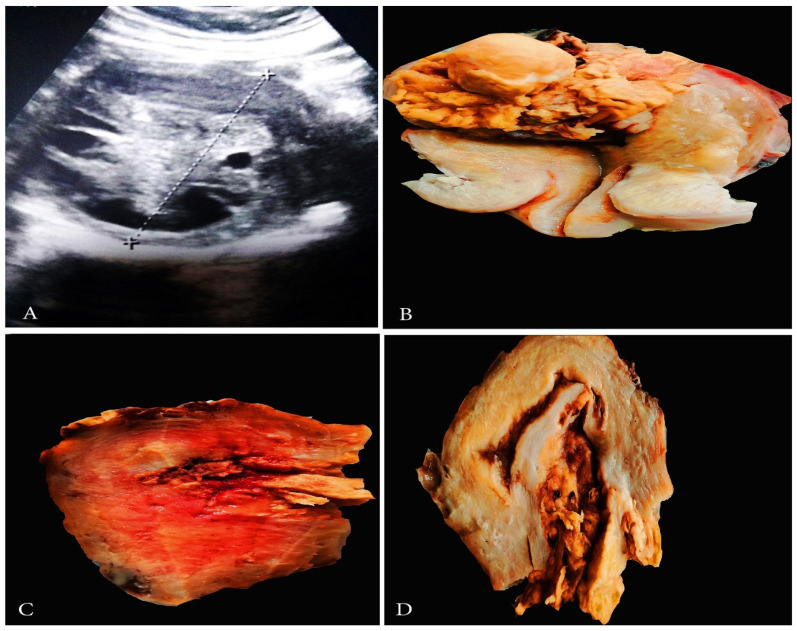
Ultrasonographic and grossly features of LGESS. (A) Abdominal ultrasonography of LGESS; (B–D) Gross appearance of LGESS.
